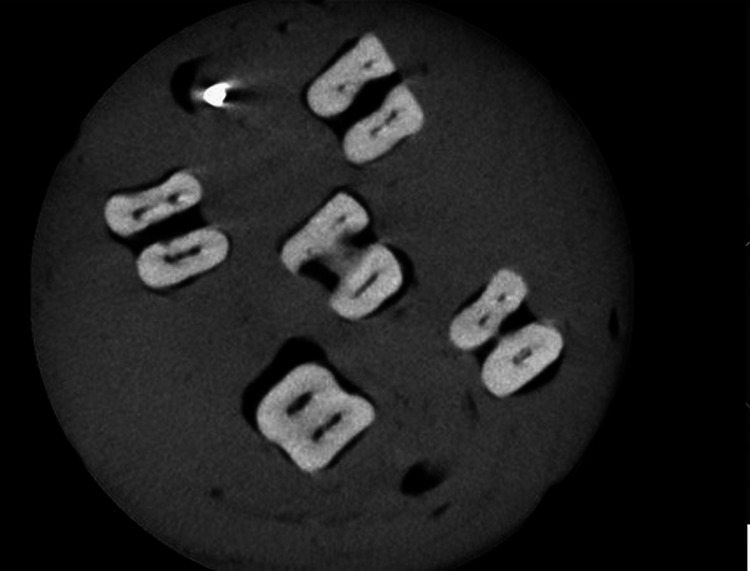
Cone beam computed tomography images of the root canal
